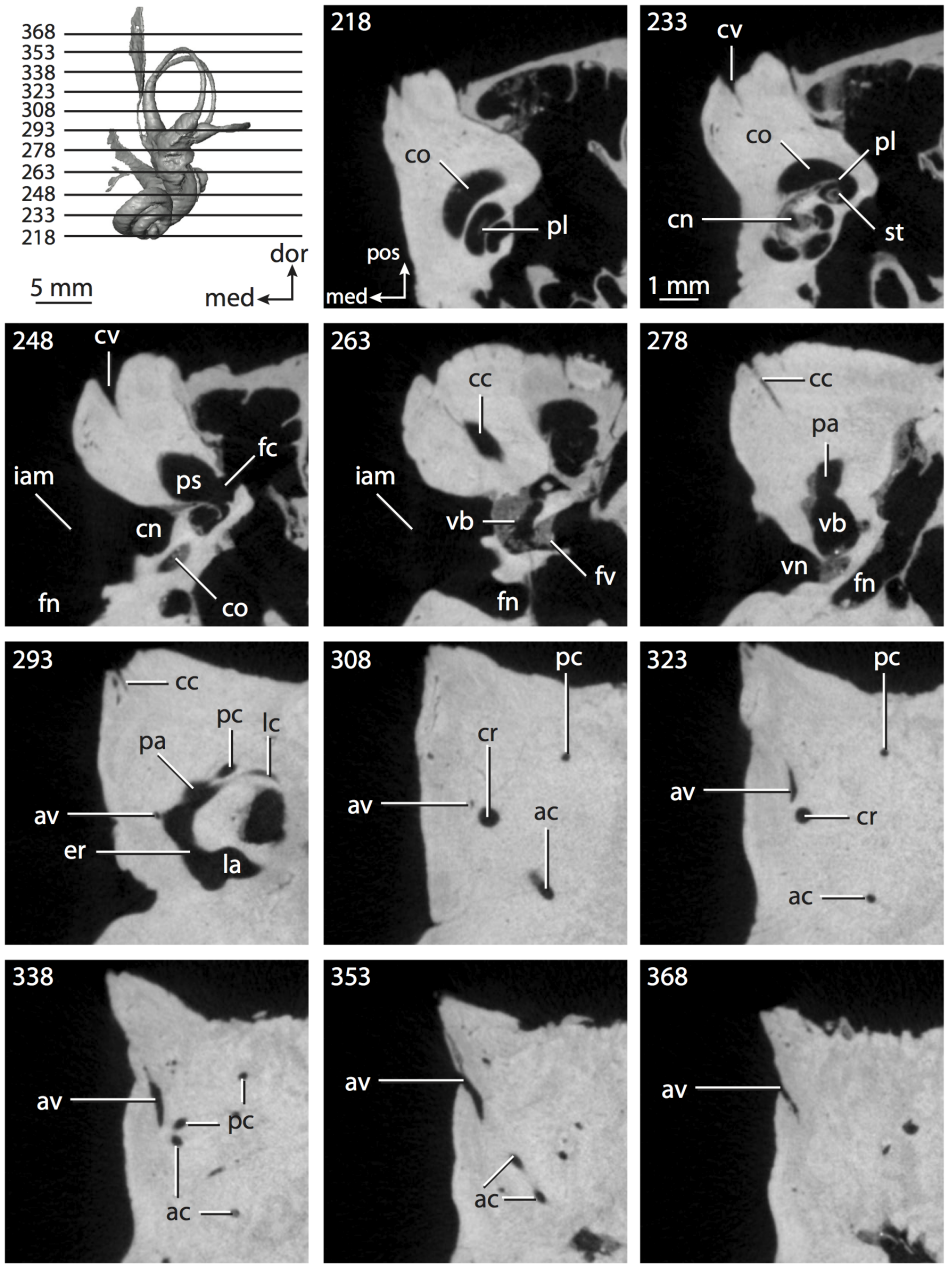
CT slices through ear region of*Equus caballus.* Abbreviations listed at the end of the Materials and Methods section.
